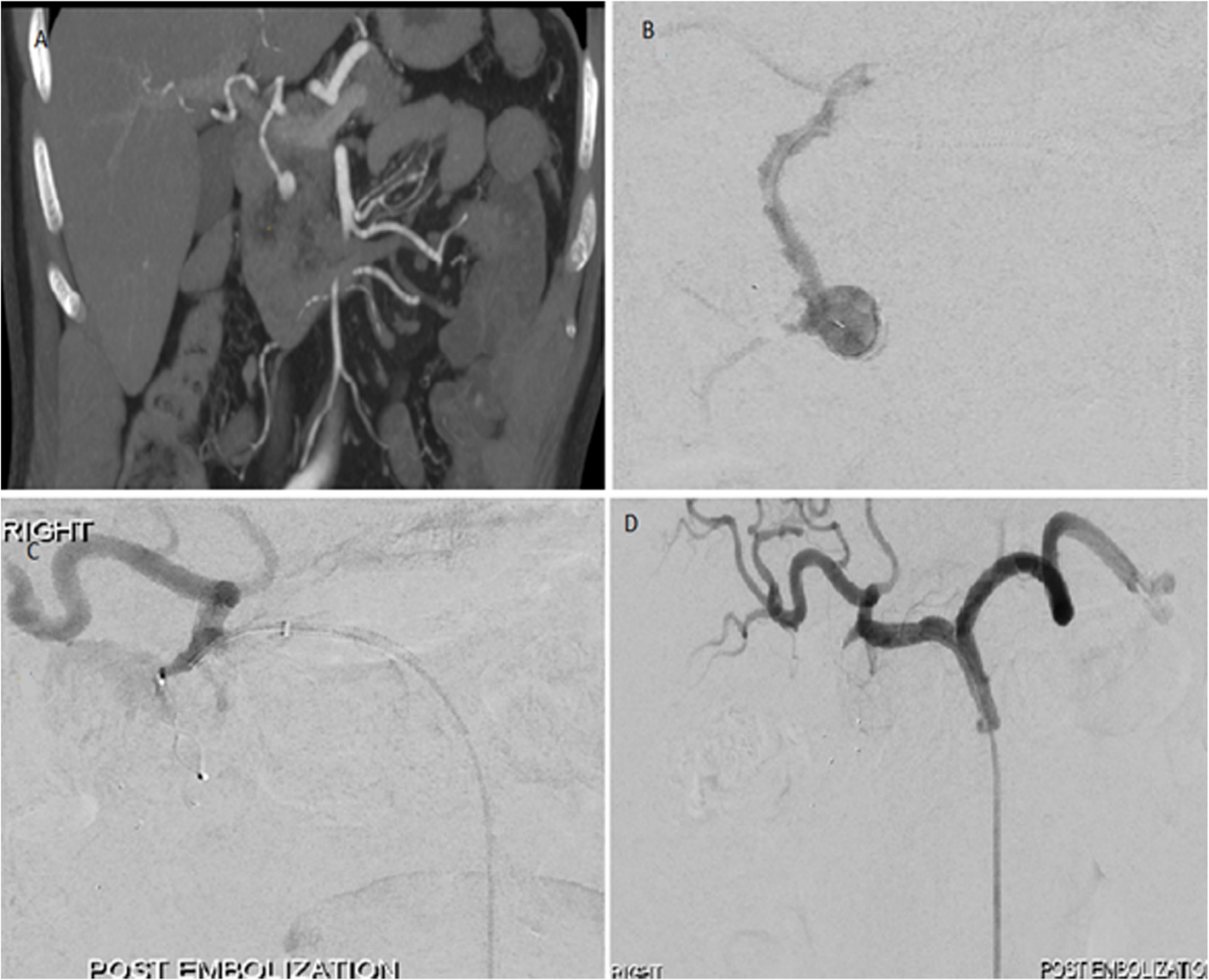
Embolisation of gastroduodenal artery pseudoaneurysm. a. CT angiogram showing gastroduodenal artery pseudoaneurysm surrounded by hematoma. b Selective angiogram of the gastroduodenal artery demonstrating the lesion. c Embolisation of the gastroduodenal artery pseudoaneurysm by trapping technique with 6.5 mm and 5 mm diameter microvascular plugs distally & 7 mm diameter Amplatzer IV plug proximally. d Final angiogram showing complete exclusion of the pseudoaneurysm from the circulation
